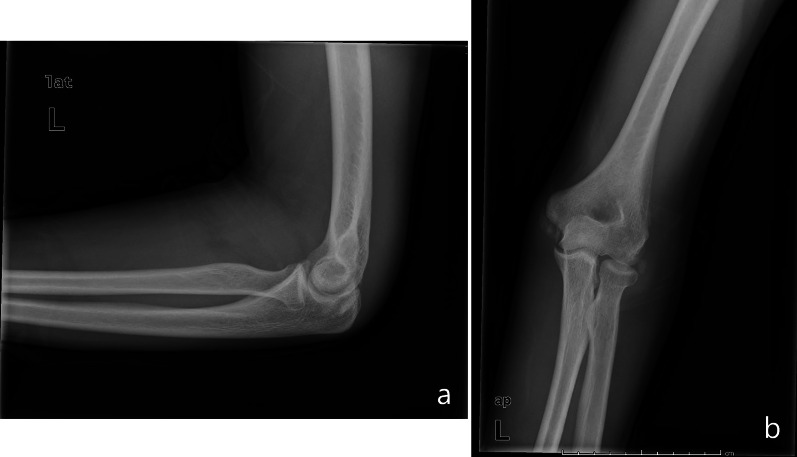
Heterotopic ossifications on X-ray examination. X-ray examination with heterotopic ossifications findings around both lateral and medial condyles (a, b)
